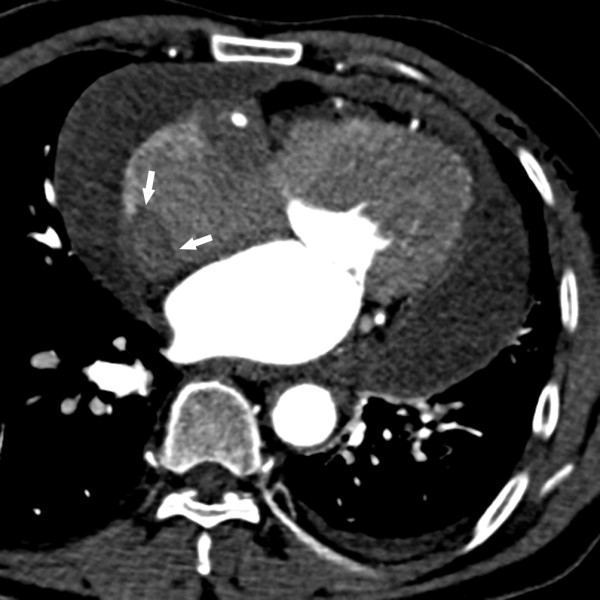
A contrast-enhanced computed tomography image demonstrating an irregularly shaped, 25.0-mm right atrial mass extending along the right atrial free wall (white arrow); minor left-sided pleural effusion; slight pleural thickening of the right side; and diffuse pericardial effusion.
